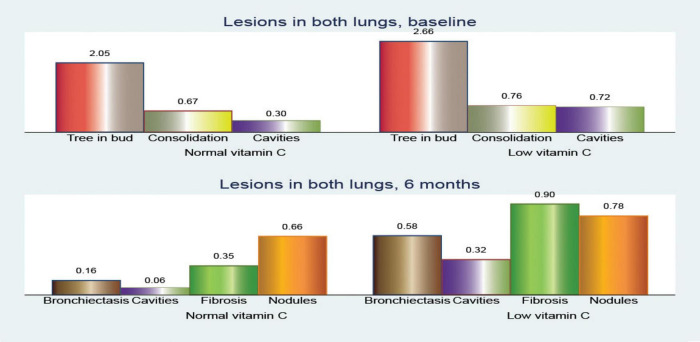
Comparison of pulmonary computed tomography (CT) lesions at baseline and after 6 months of treatment.
